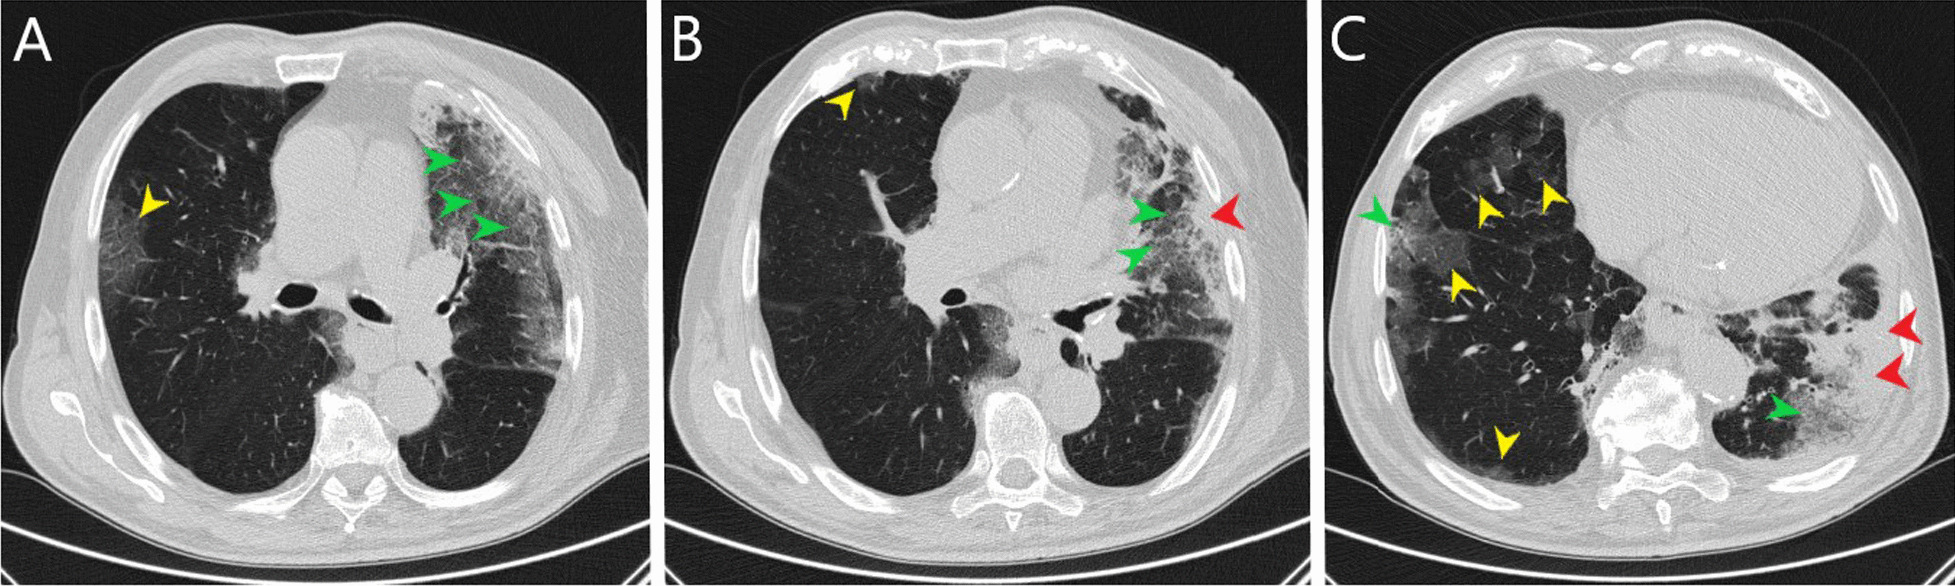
Chest high resolution computed tomography (A–C lung window, axial scans): bilateral, predominately peripheral, ground-glass opacities (yellow arrowheads), crazy paving (green arrowheads) and consolidations (red arrowheads) especially in left lower lobe
